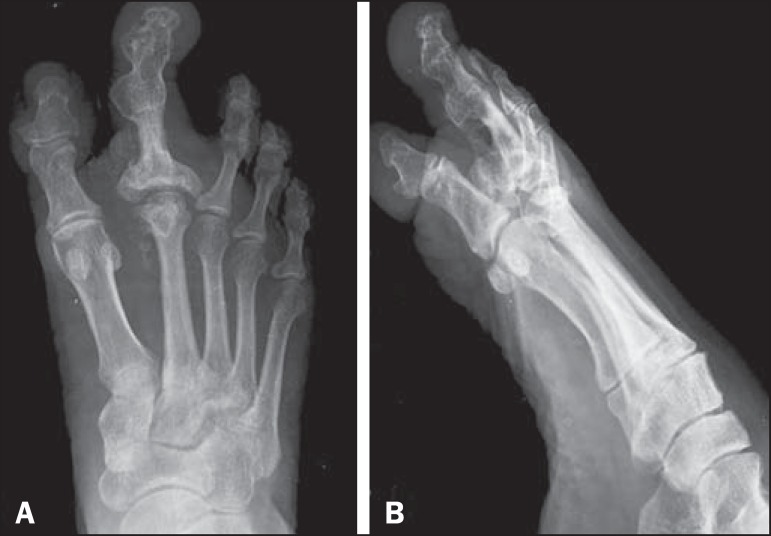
Plain radiographs, in the anteroposterior and oblique planes
